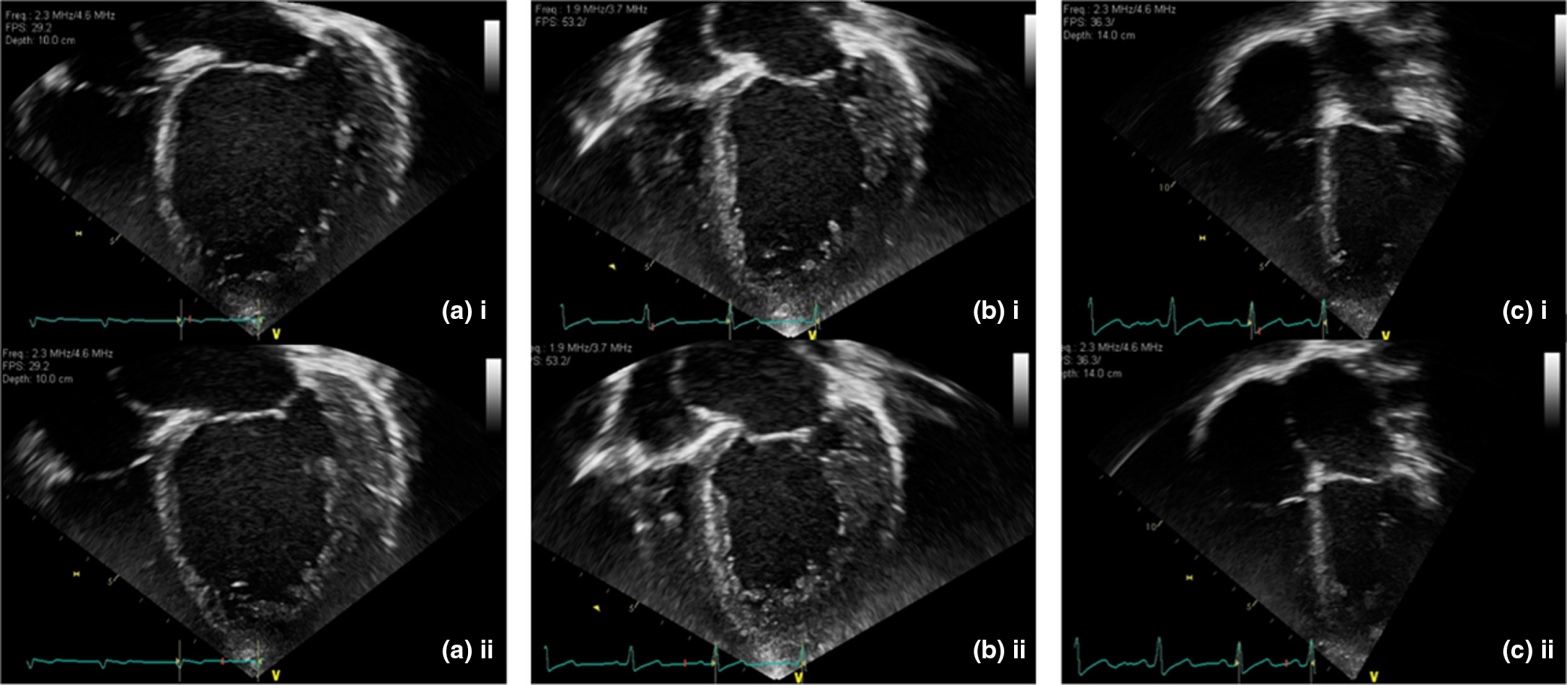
Apical four‐chamber transthoracic echocardiogram. (a) ECHO at time of diagnosis, (b) after 8 weeks of carnitine supplementation, (c) after 2 years of carnitine supplementation. (i) end‐diastole, (ii) end‐systole.
